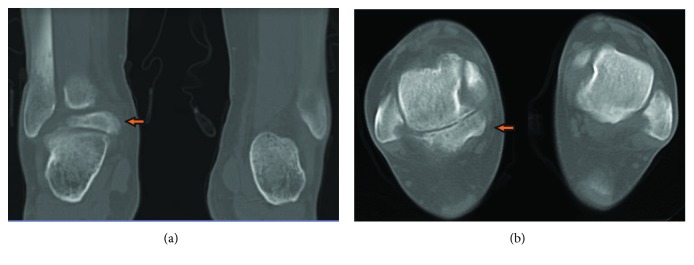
Comparative CT scan showing posterior bone process, displacing into the medial side (arrow) in both coronal (a) and axial (b) view.
